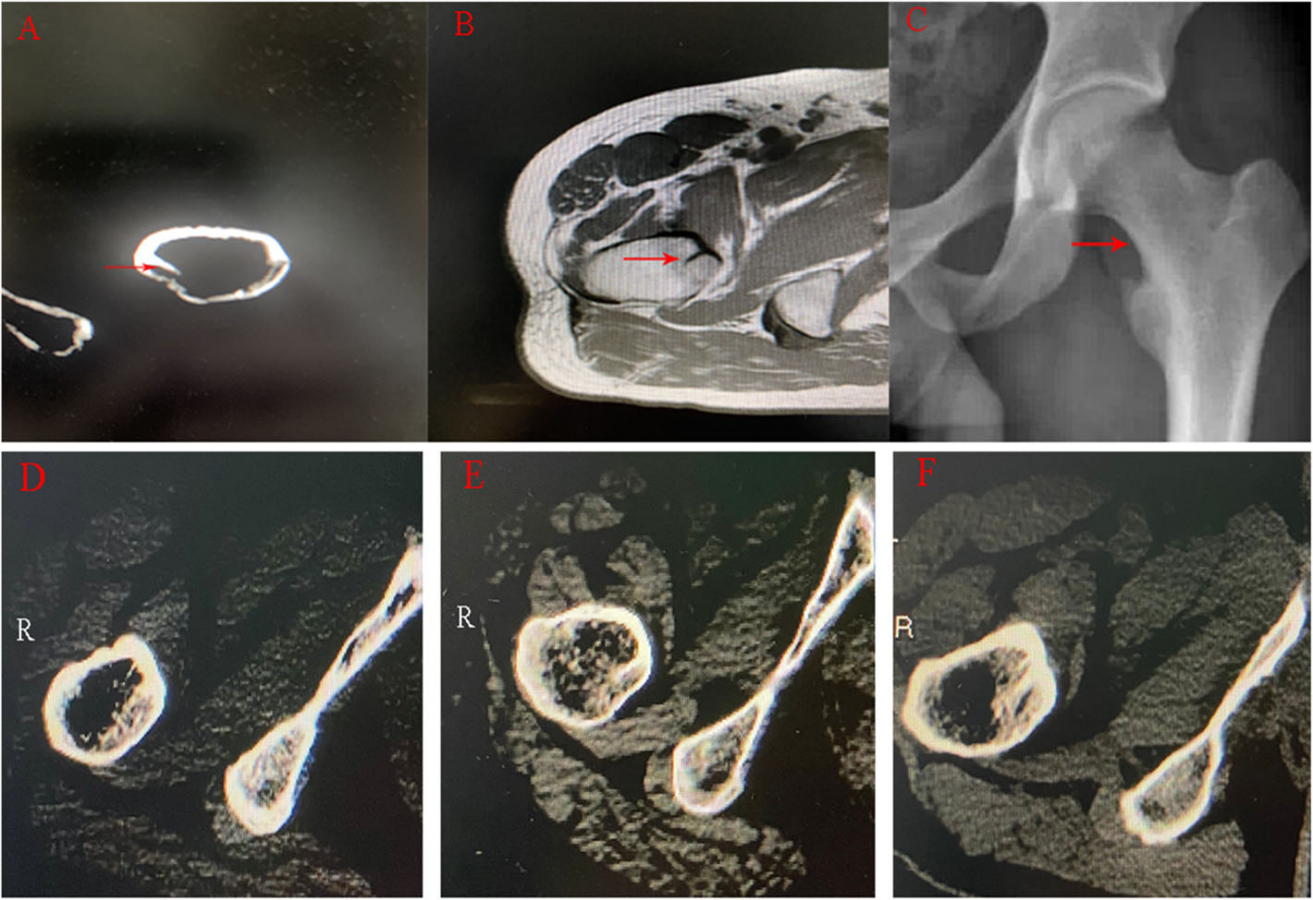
a The spur type of the calcar femorale can be seen in cross-sectional computed tomography images (red arrow) b The calcar femorale has a low signal in T1 weighted magnetic resonance images (red arrow) c The Adams arch (red arrow) is often confused with the calcar femorale. These three cross-sectional computed tomography images are from different individuals, the calcar femorale is classified into three types according to its length and thickness. d ridge-type: the calcar femorale is short and thick. e spur-type: the calcar femorale is longer than ridge-type and like a spur. f septum-type: the calcar femorale is thin and long
